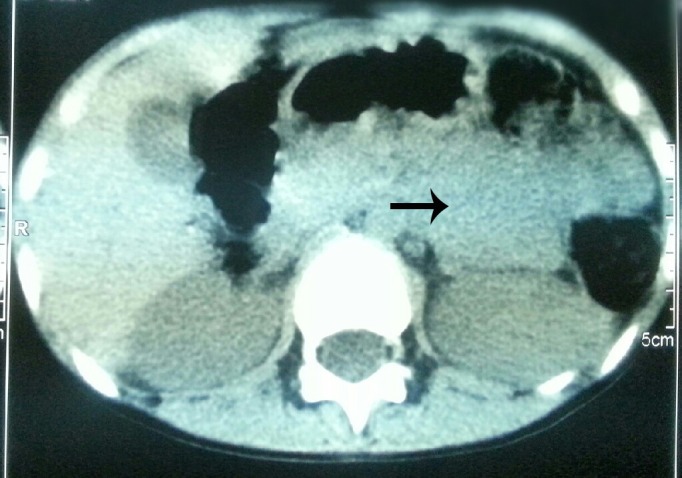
Figure 1: CT scan showing suprarenal mass (arrow).
